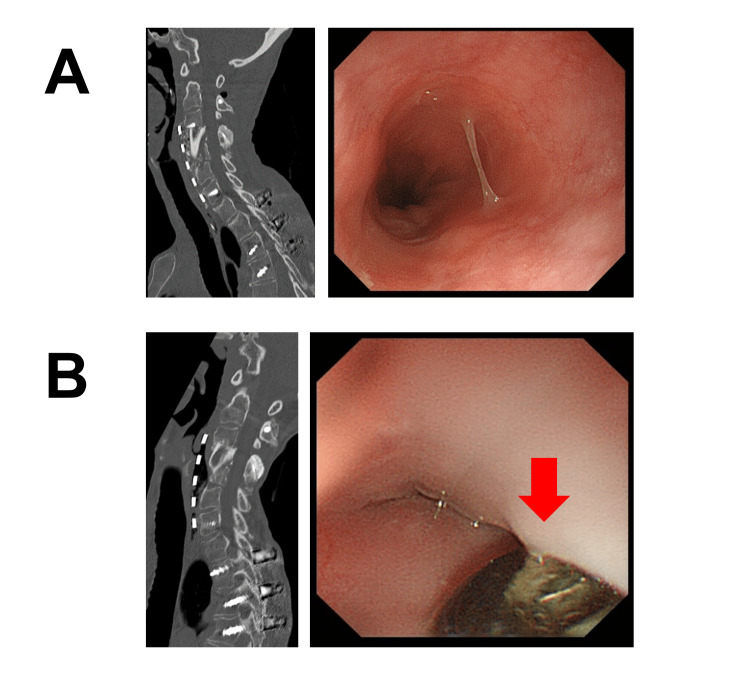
Follow-up CT and UGE Images at one year (A) and over three years (B) after surgery.
